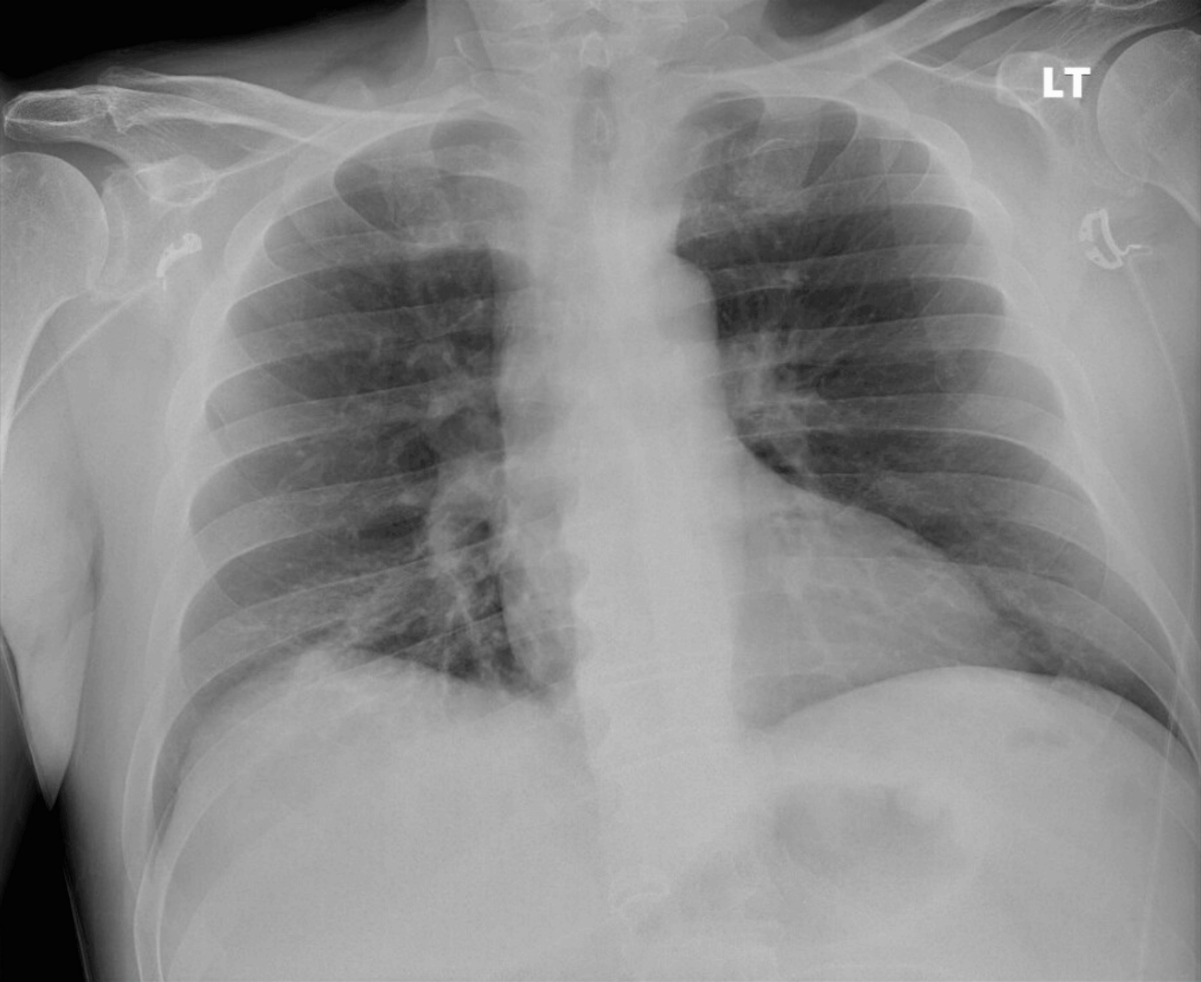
CXR taken on the day of admission showed no significant intrathoracic abnormality, with normal bilateral lungs and a normal-sized heart. This was confirmed by the radiological read and reviewed by the primary team physician. CXR: Chest X-ray
